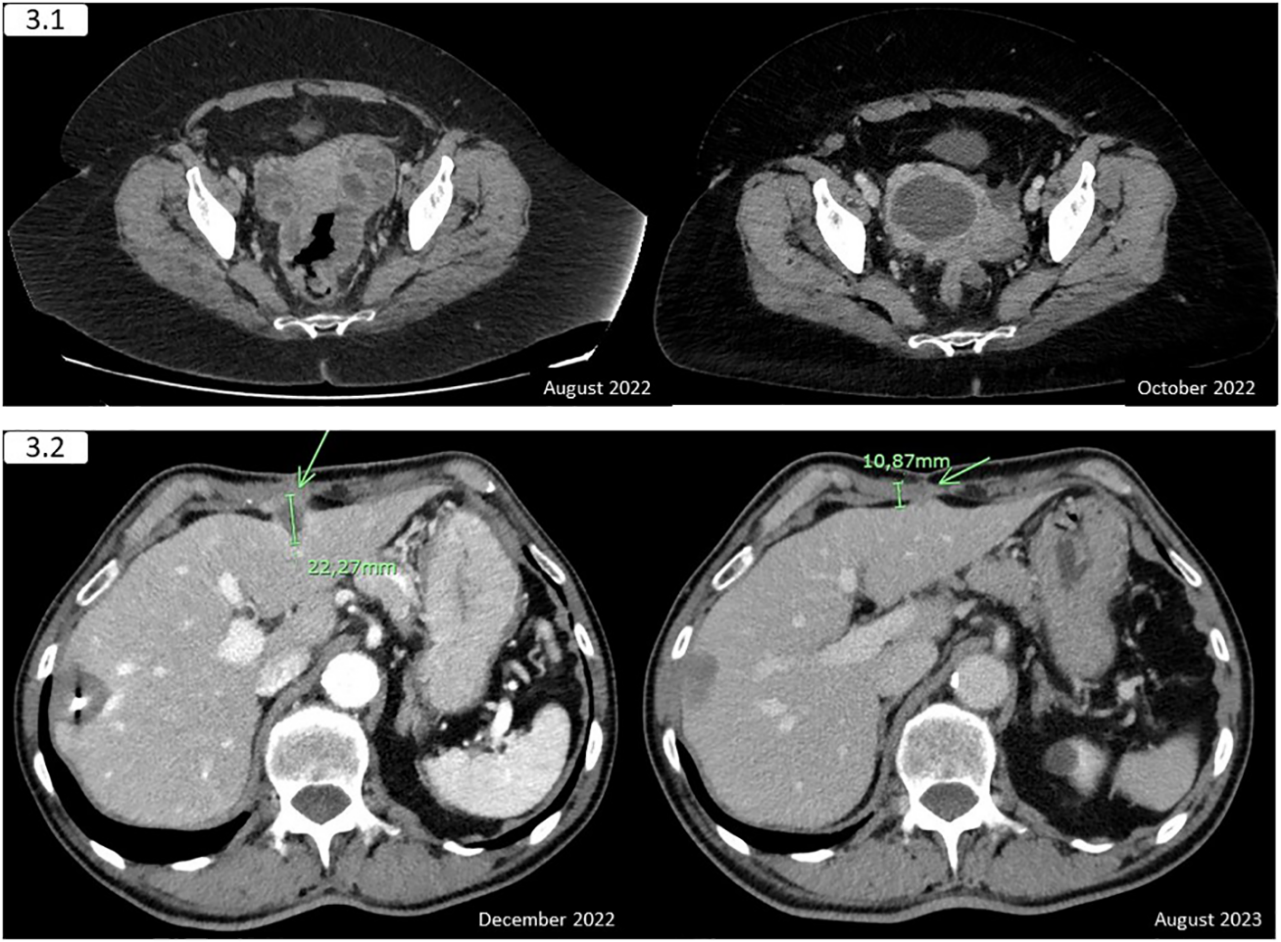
3.1 (Case report 3) - The interval CT scan revealed an amelioration of the rectal and adnexal infiltration, with the apparition of a pyometra or a right-annex abscess that provokes an ipsilateral ureterohydronephrosis. A decrease in the abdominopelvic nodes was also observed. 3.2 (Case report 4) - The previously treated with radiofrequency 33-millimetre liver lesion in the right hepatic lobe showed no changes and a remarkable decrease is appreciated in the segment III metastasis, remaining the signs of the hepatic capsule and anterior abdominal wall infiltration. The green arrow indicates the location of the infiltrating liver lesion.
